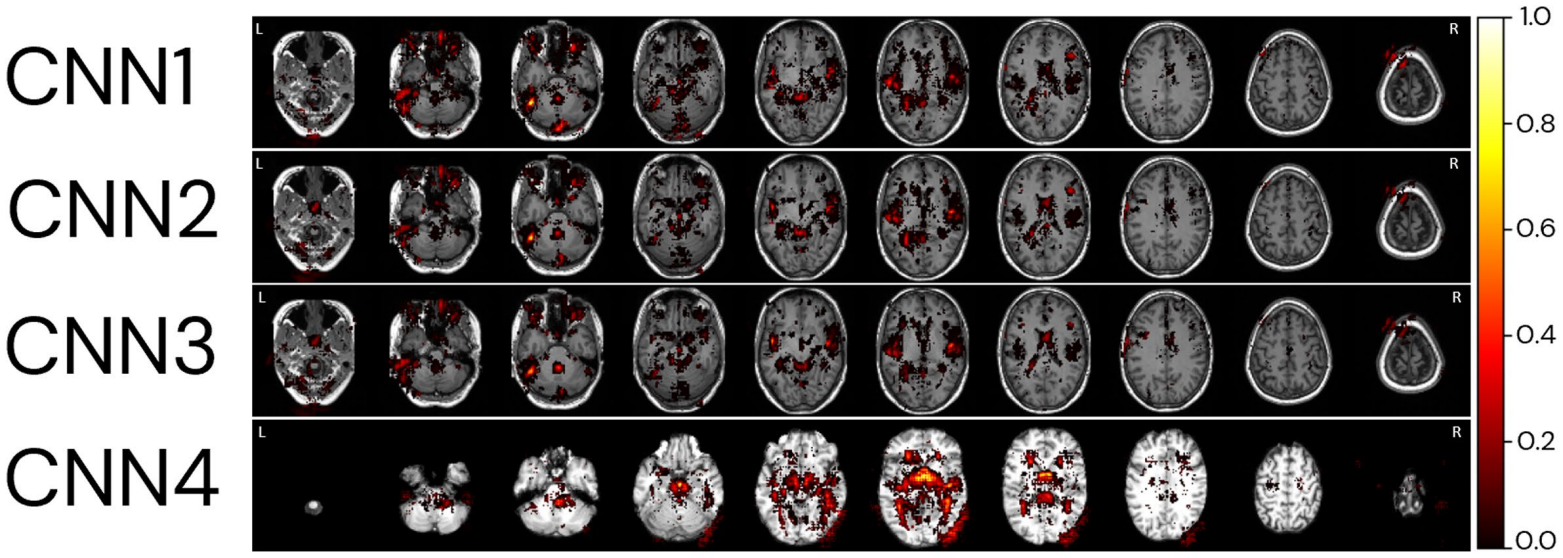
Relevant regions for age prediction. The absolute values of the salience maps for each model were averaged through the whole MRI sample and normalized between 0 and 1 for better visualisation. The SmoothGrad method was used to generate all salience maps. Overlayed salience map colours are normalized for each individual between 0 and 1.
